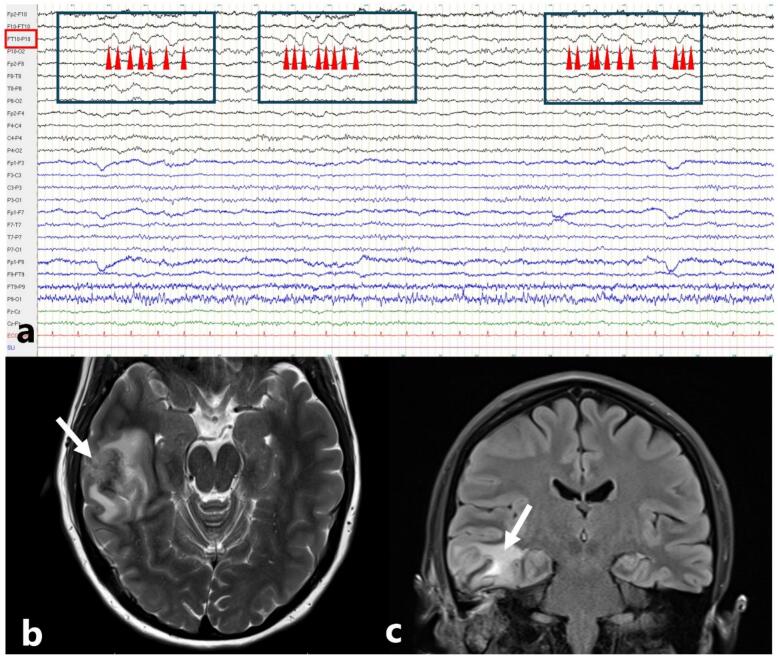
Polymorphic delta activity in a patient with epilepsy consecutive to temporal abscess (a) Scalp EEG displaying 3 instances of right, polymorphic focal slowing centred over temporal leads (FT10-P10). Note the inhomogeneous shape of delta activity across the 3 boxes. Arrowheads point towards zero-crossings; as seen, these are not regular across time, reflecting more heterogenous frequency content than the activity depicted in Fig. 3, Fig. 4. Note also the more variable amplitude of polymorphic delta activity. (b) Axial T2 and coronal FLAIR MRI exhibiting the right temporal abscess.
